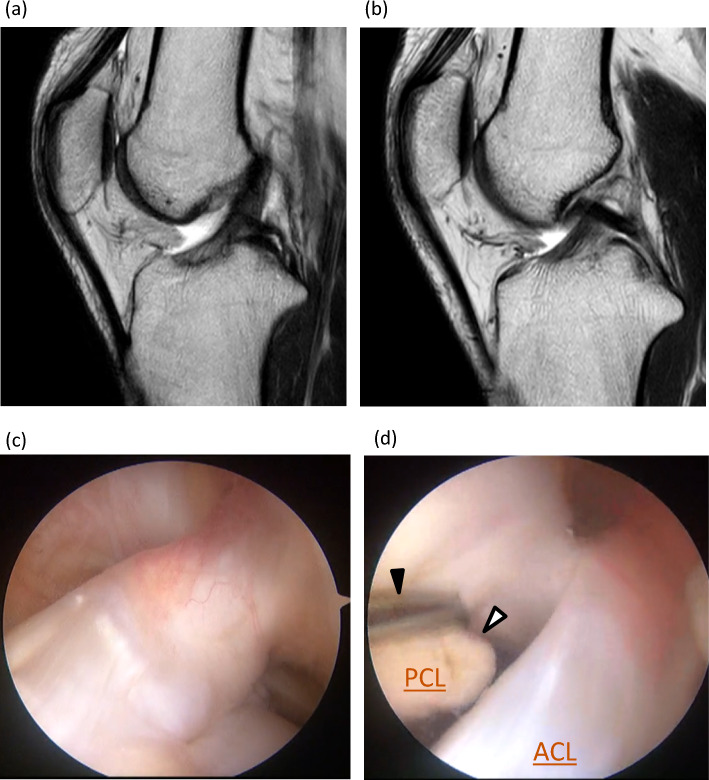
A representative case of chronic PCL injury; a 26-year-old man, injured 3 years ago when he sprained his left knee while snowboarding. a Sagittal MRI image of the left knee; the ACL appears to be injured. b There is continuity in the PCL. c Arthroscopic findings; the ACL was deflected, but when the tibia was pulled forward, tension was normal and there was no injury. d Probing PCL; PCL was partially injured. The black arrowhead represents the tip of the probe, and the white arrowhead represents the torn end of the PCL from the femoral side. ACL, anterior cruciate ligament; PCL, posterior cruciate ligament; MRI, magnetic resonance imaging
